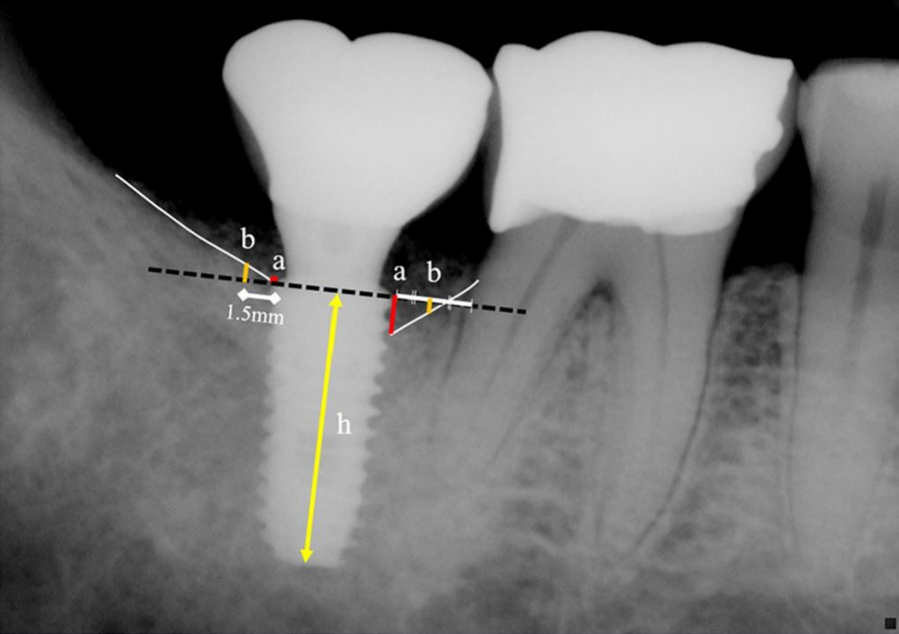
On periapical radiographic image, fixture length (*h*) and marginal bone levels at direct bone–implant contact points (*a*) and distant crestal points (*b*) were measured using image processing program (Image J, National Institute of Health). Using measured lengths, actual marginal bone level was calculated by proportional formula [*H*:*X* = *h*:*a*(or *b*)] (*H* = known actual length of implant fixture, *X* = actual marginal bone level)
